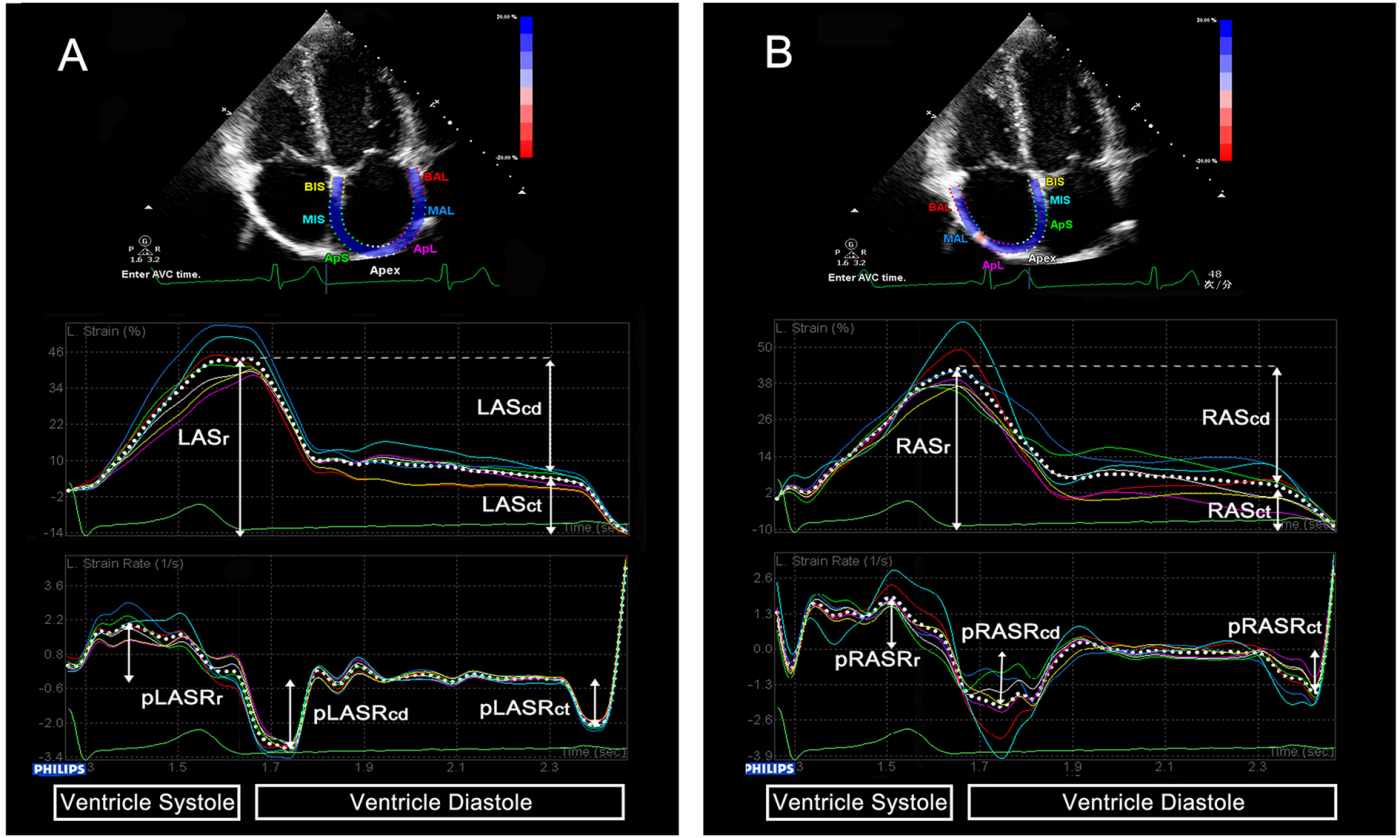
Two-dimensional speckle-tracking echocardiographic assessment of atrial function. Measurement of left atrial (A) and right atrial (B) reservoir, conduit and contractile function by strain and strain rate curves. *LA* left atrial, *RA* right atrial, *Sr* strain during the reservoir phase, *Scd* strain during the conduit phase, *Sct* strain during the contractile phase, *pSRr* peak strain rate during the reservoir phase, *pSRcd* peak strain rate during the conduit phase, *pSRct* peak strain rate during the contractile phase
